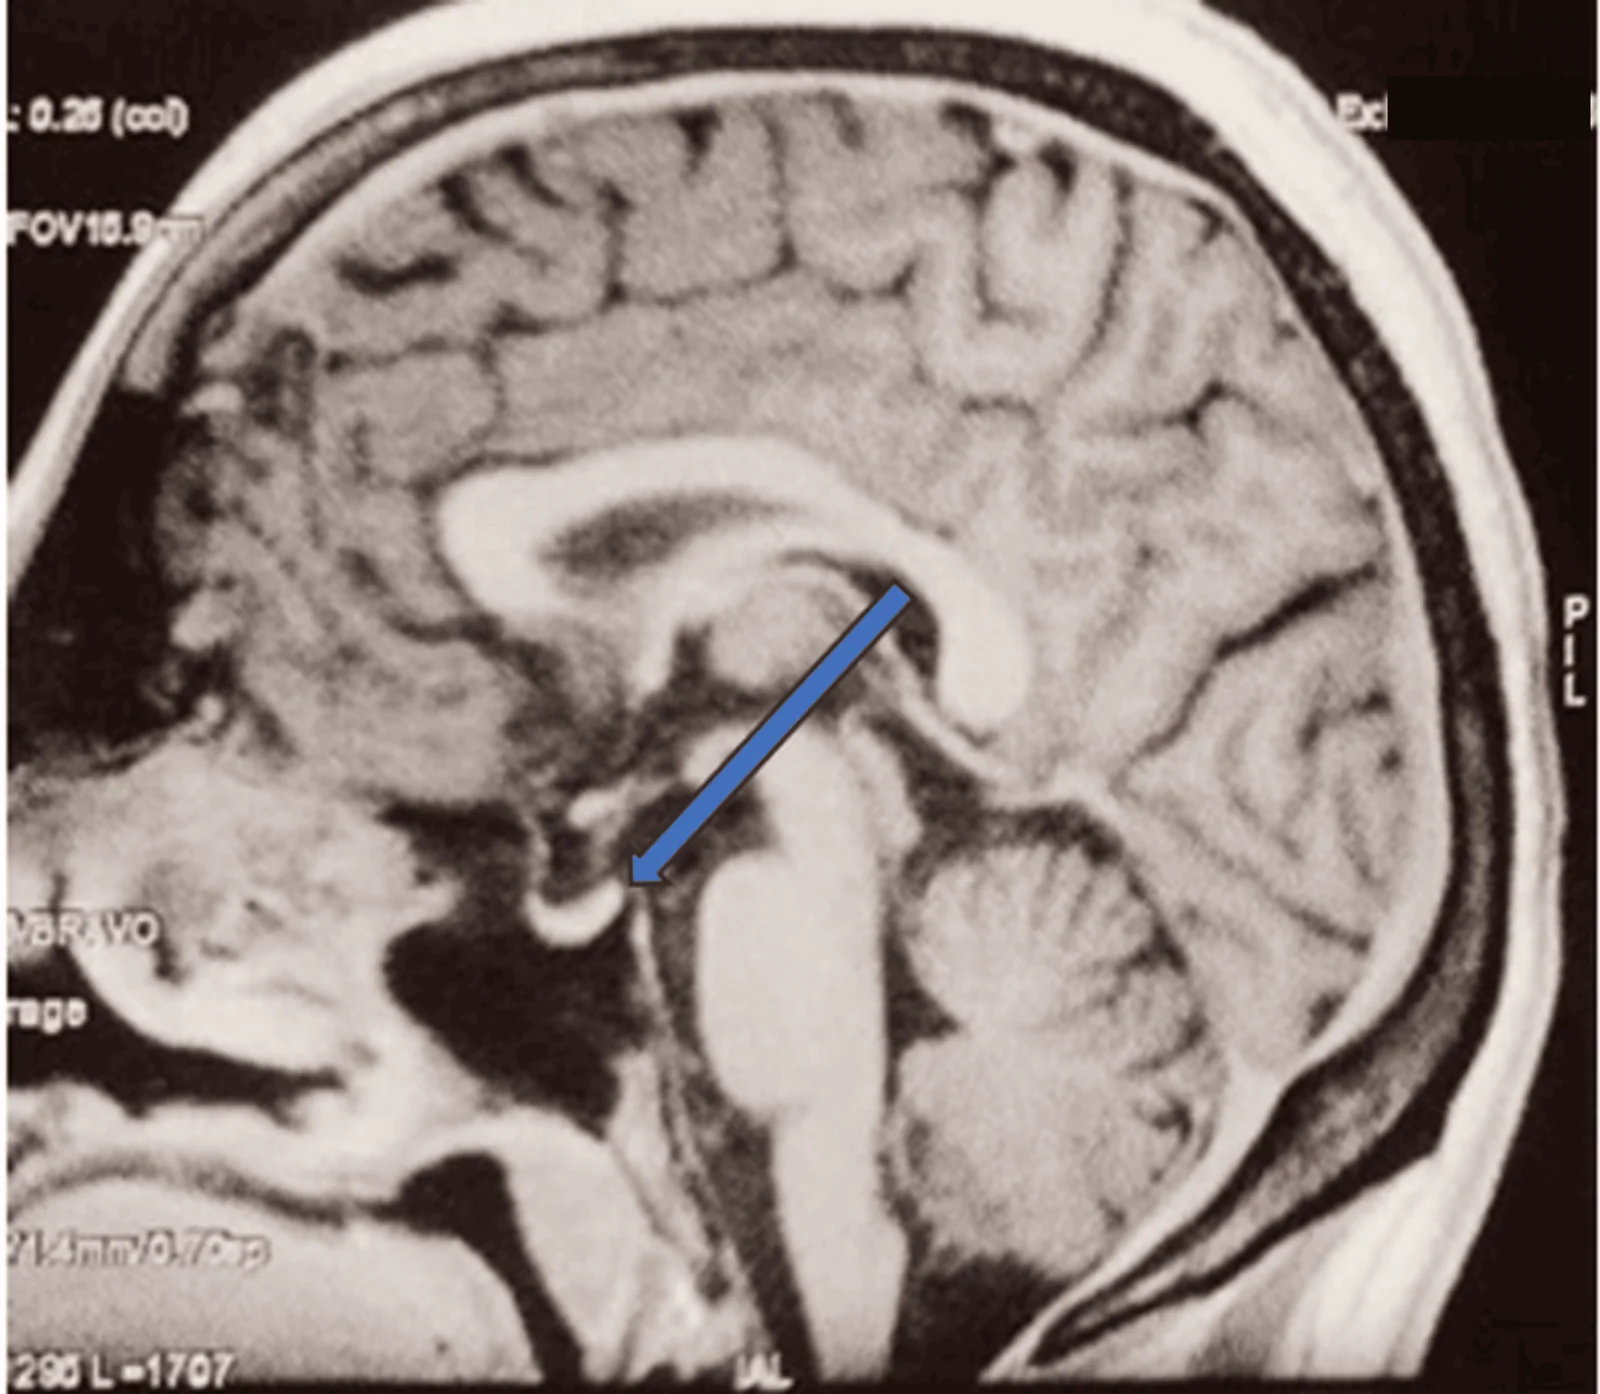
Sagittal T1-weighted MRI of the hypothalamic-pituitary region demonstrating an empty sella turcica, characterized by a flattened pituitary gland along the sellar floor with cerebrospinal fluid filling the sella, consistent with a structural etiology of partial central diabetes insipidus. MRI: magnetic resonance imaging
